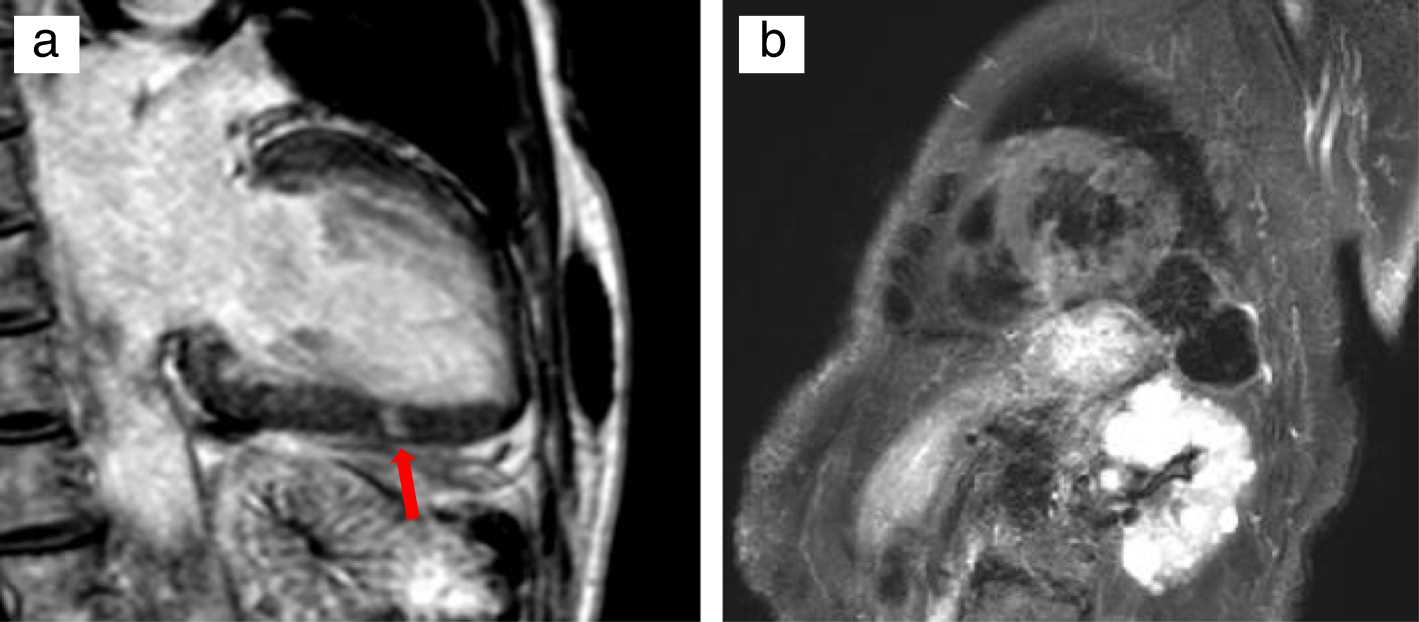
Contrast-enhanced cardiac magnetic resonance image taken 5 months after hospital discharge showing spotty late gadolinium enhancement in the middle inferior wall (a) and no high-intensity area in the T2-weighted image (b)
